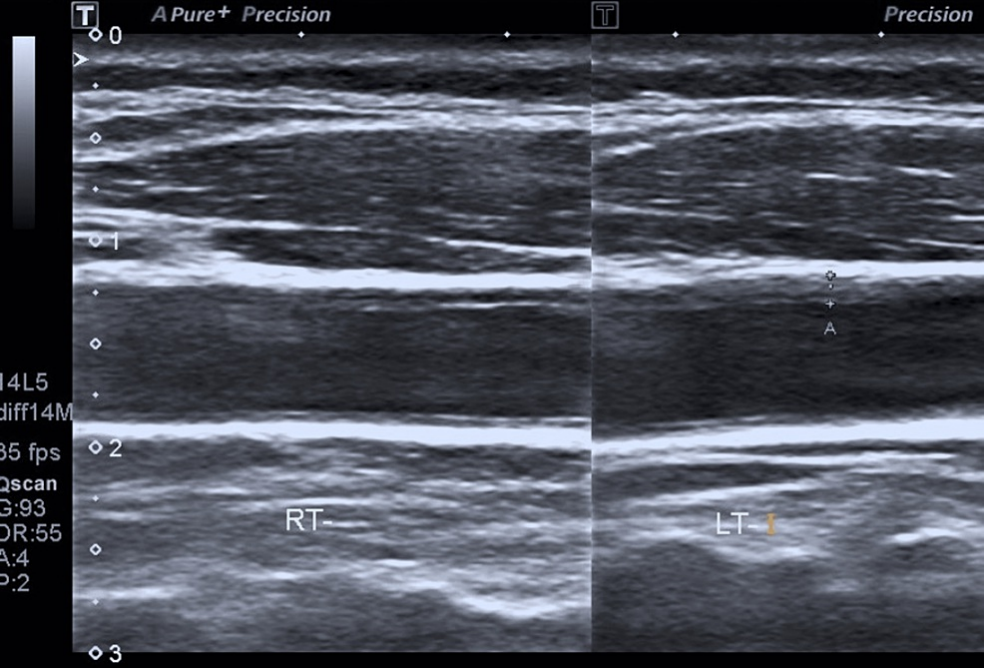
Neck ultrasonography revealing thickened vessel wall (1.4 mm) of the left common carotid artery.
